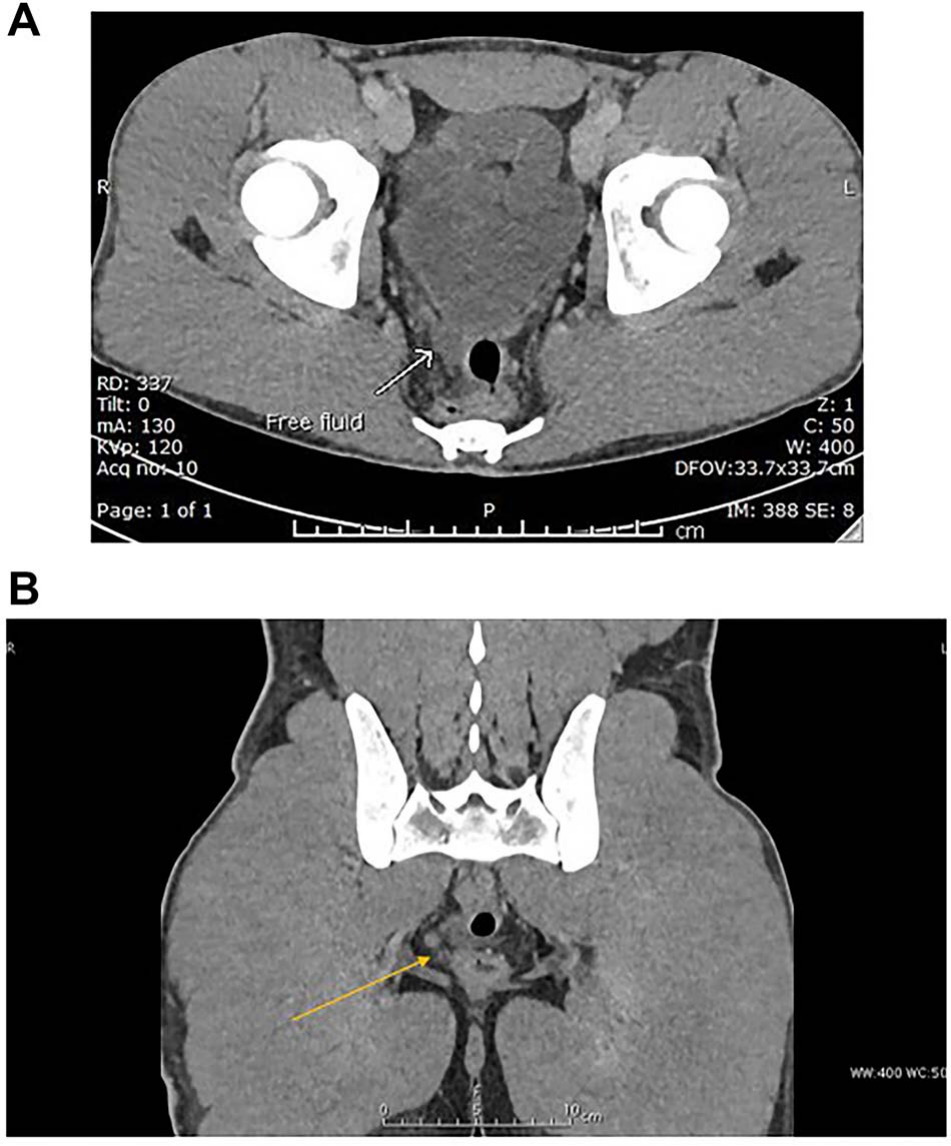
(A) Venous phase axial CT scan showing minimal amount of free fluid in the Douglas pouch (retro-vesical). (B) Venous phase coronal CT scan showing minimal amount of free fluid in the Douglas pouch (retro-vesical).
